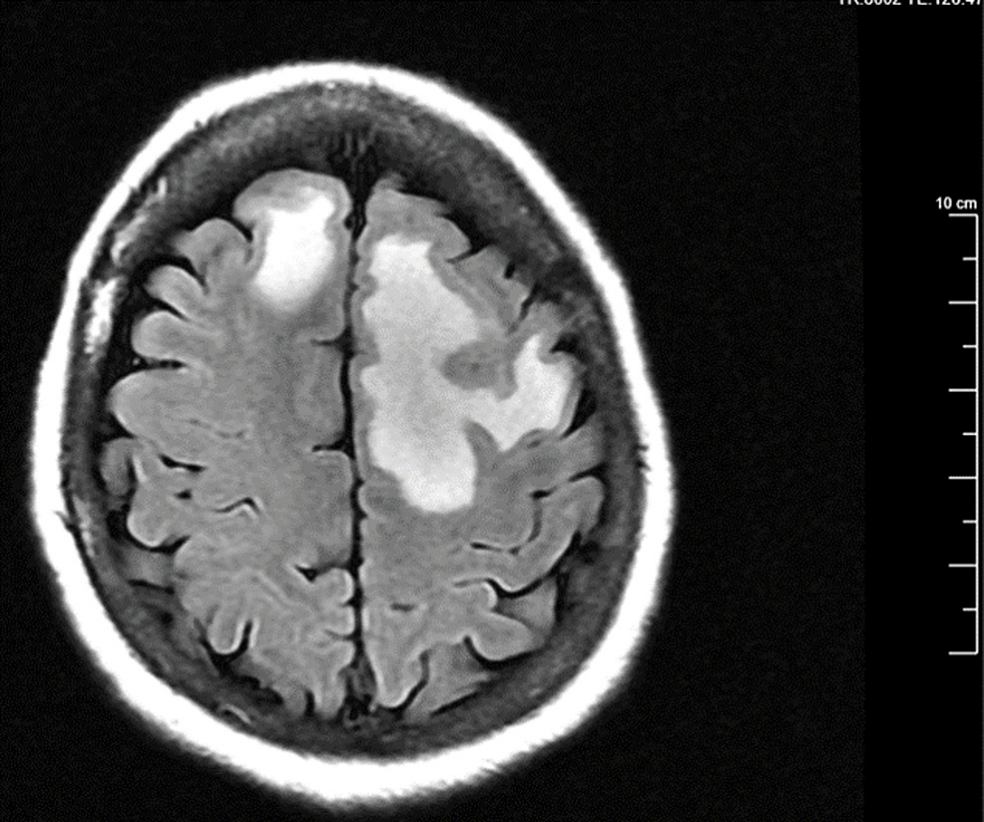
MRI of the brain metastases demonstrates two areas of intra-axial enhancing lesions in the left precentral gyrus and the right frontal lobe with restricted diffusion. MRI: magnetic resonance imaging
